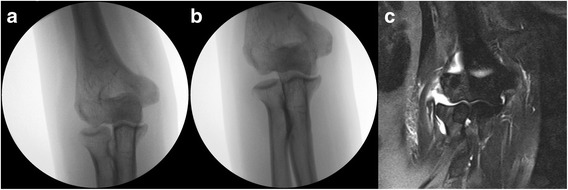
a Intraoperative fluoroscopy image of a 53-year-old woman (patient #1). b Widening of the radiocapitellar joint was identified 2.3 mm in the varus stress view. c T2-weighted coronal magnetic resonance image showed rupture of the lateral collateral ligament and tear of the extensor tendon origin
